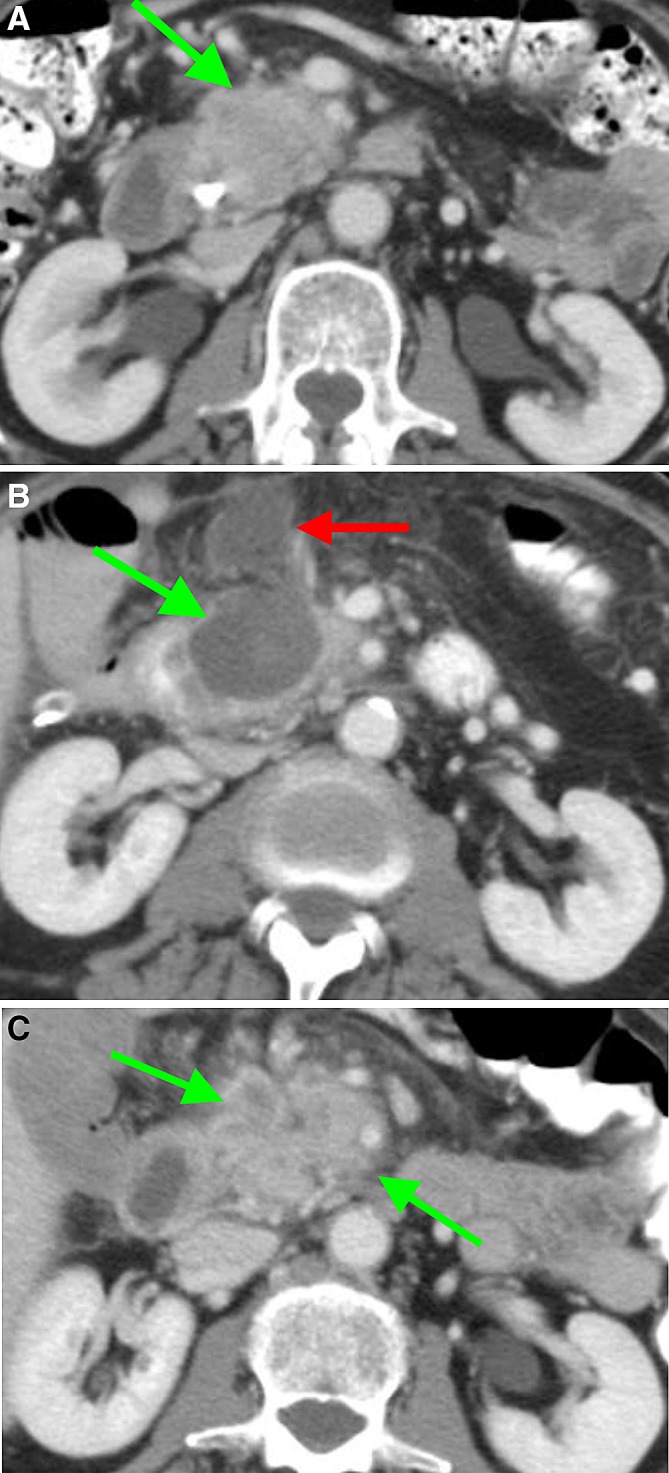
CT-images in the axial plane, of a 74-year-old woman with A a ductal adenocarcinoma in the head of the pancreas (arrow) on the pre-RFA scan, showing 90°–180° circumferential involvement of the superior mesenteric artery. B One-week post RFA, a sharply demarcated ablation zone is visible as a heterogeneous area (arrow). Moreover, the ablation zone extends ventrally outside the pancreas contour and a fluid collection is present in the transverse mesocolon. C Tumor replacing the ablation zone (arrow) at 3 months post-RFA. Note the inhomogeneous enhancement, the mass effect and the increasing size and involvement of the vessels over time
